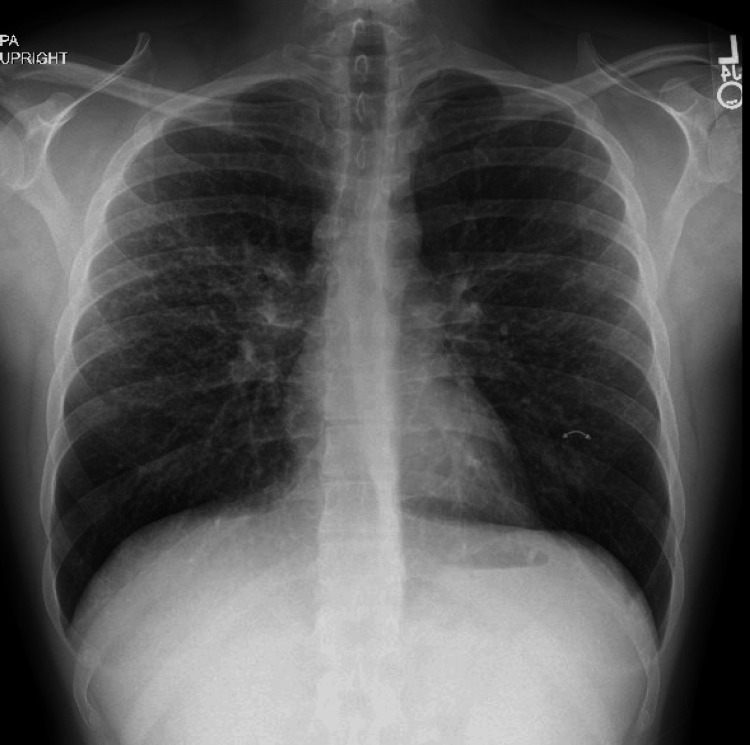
Chest X-ray of the patient suggesting diffuse pulmonary sarcoidosis
